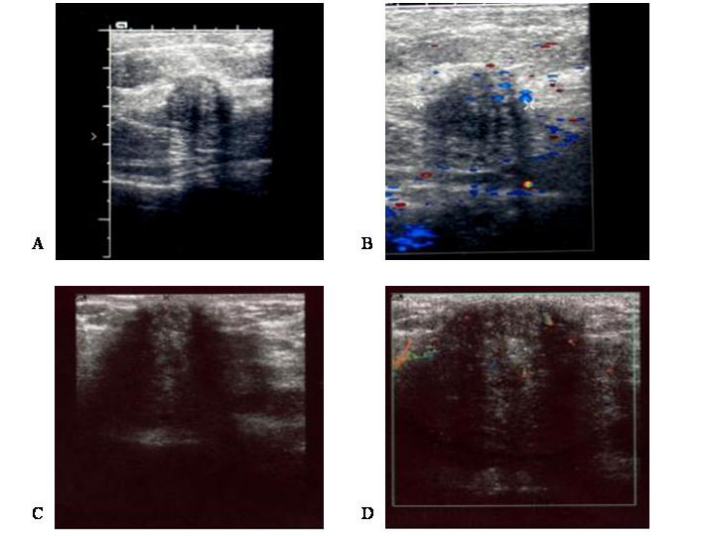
2-D and power Doppler sonographic views of scar endometriosis nodules. Using 7.5 MHz transducers, scar endometriotic lesions appeared hypoechoic, with internal echoes on 2-D ultrasound (A, C), and with internal vascularity on power Doppler (B, D). A and B: Case 1; C and D: Case 2.
